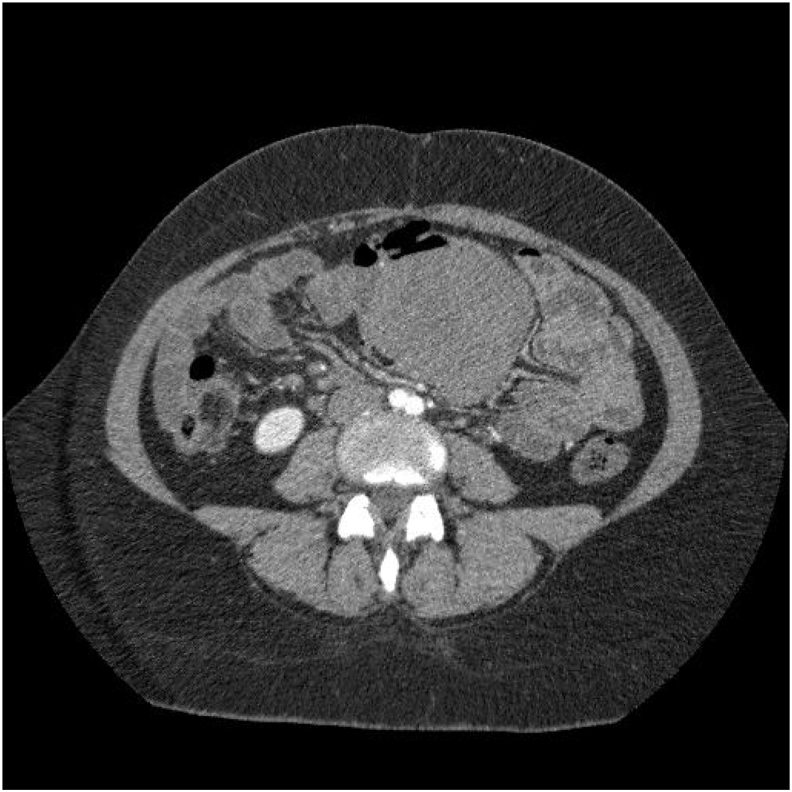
Abdominal computed tomography showing the 23 × 11 × 10 cm mesenteric desmoid tumor with small intestine compression and free intraperitoneal air.
